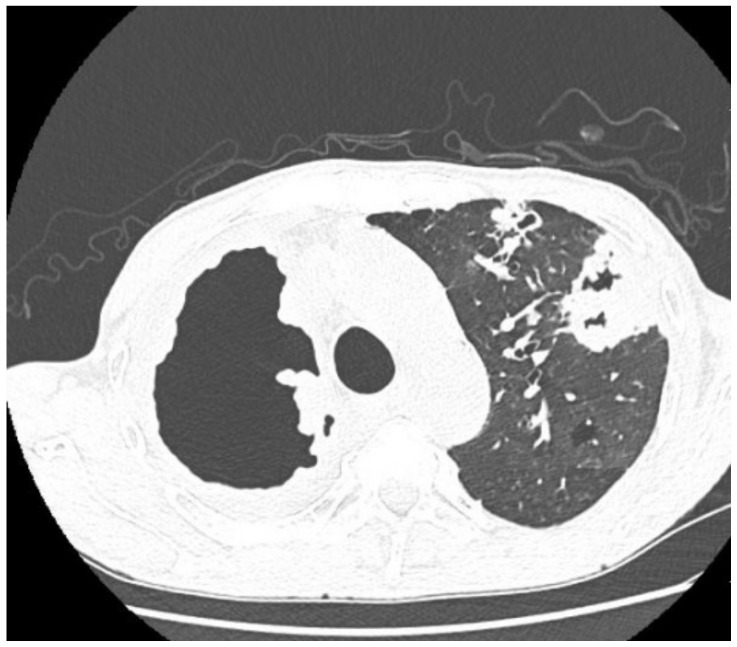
CT scan of the chest taken at the beginning of treatment 21 December 2020.
